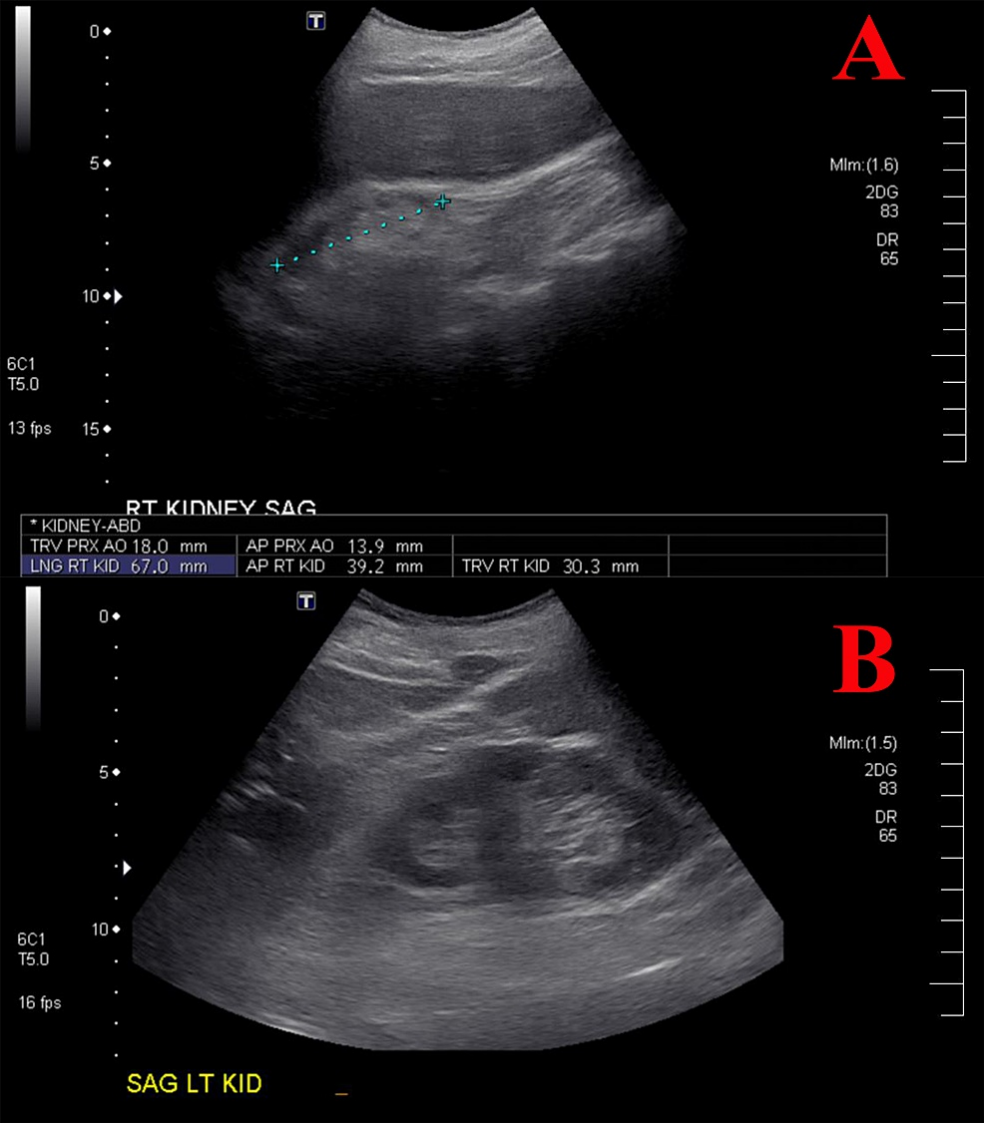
Kidney ultrasound. A: right kidney was severely atrophic (70.3 mm x 39.2 mm x 30.3 mm) with increased echo density without hydronephrosis. B: left kidney was measuring (103.7 mm x 43.9 mm x 39.3 mm) and demonstrated generalized cortical thinning without hydronephrosis.
